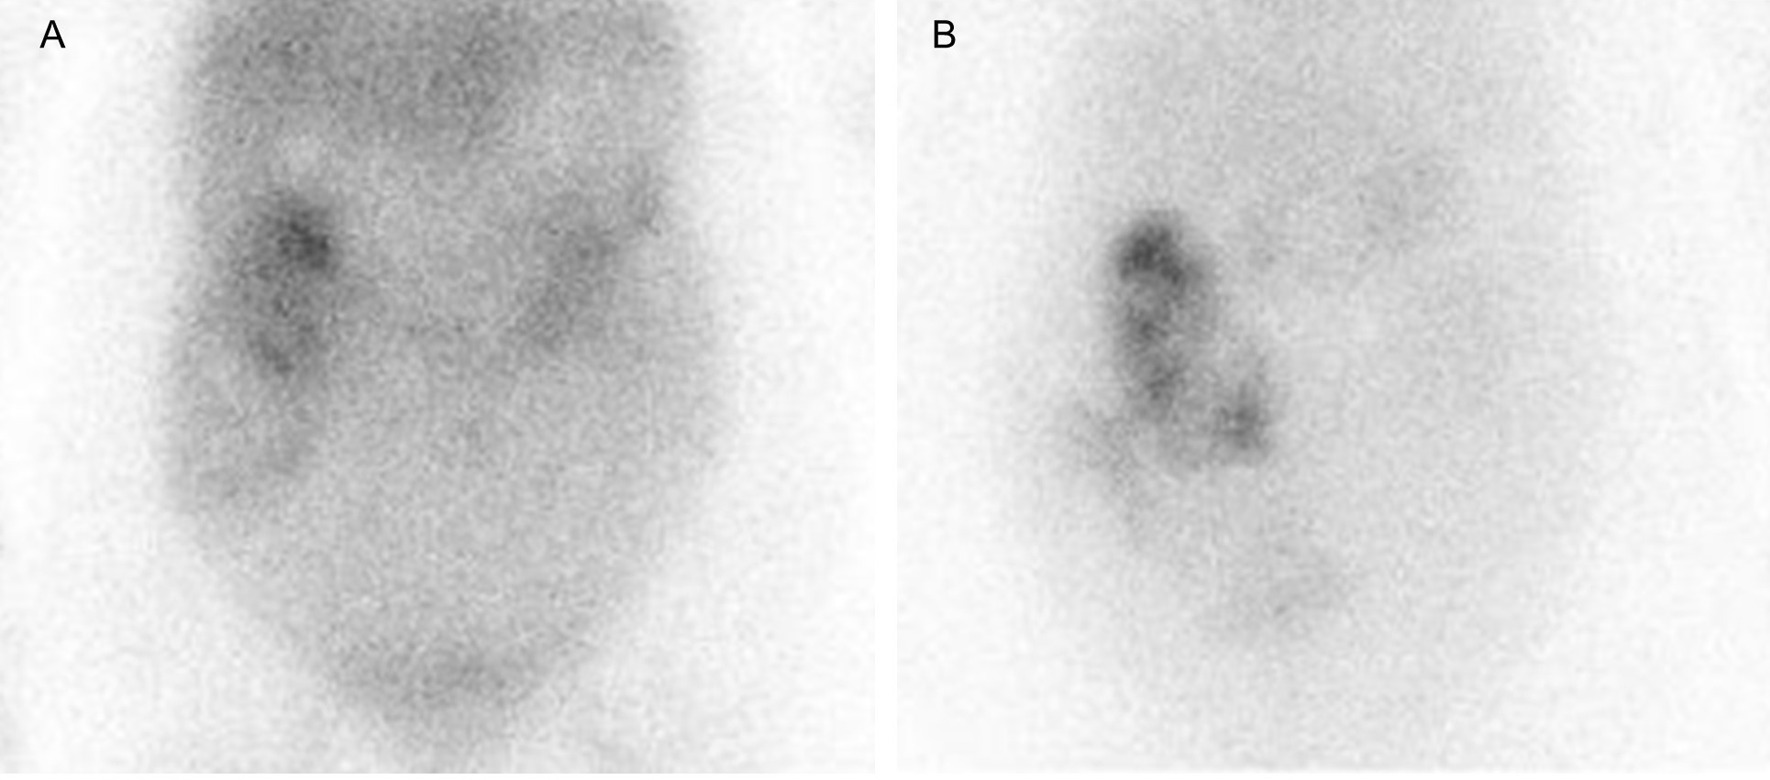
^99m^Tc-DTPA-binding human albumin scintigraphy. Protein leakage was shown from the cecum to the transverse colon
